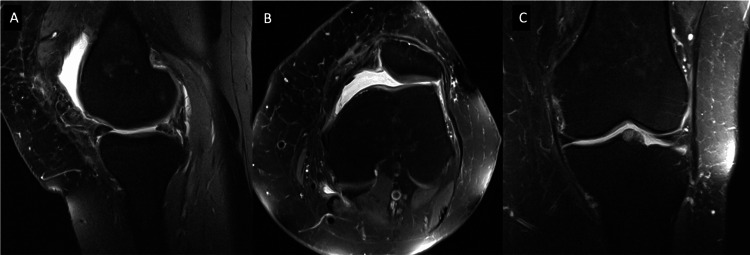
Post-operative MRI image of the knee PD FS MRI sequence: (A) sagittal, (B) axial, and (C) coronal views of the right knee demonstrating no evidence of lesion recurrence two years after surgical excision. PD, proton density; FS, fat suppression; MRI, magnetic resonance imaging
